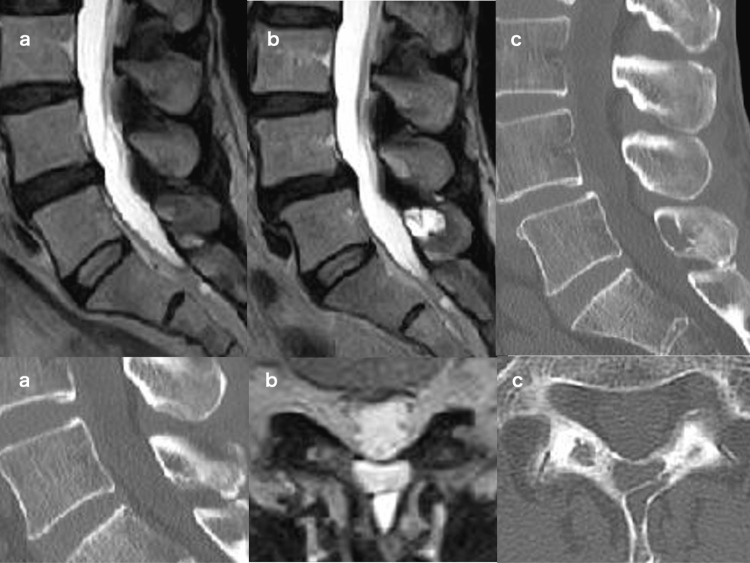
Changes in lumbar spinal images (a) Initial lumbar MRI (upper) and 3D-CT (lower) showed no more obvious findings. (b) MRI at symptom exacerbation showed L4-5 stenosis and spondylolisthesis with HLF and L5 laminar T2WI hyperintensity. Sagittal plane (upper), axial plane (lower). (c) 3D-CT at symptom exacerbation showed intramedullary low density and cavitation in the L5 laminar. Sagittal plane (upper), (lower) MRI: magnetic resonance imaging; CT: computed tomography; HLF: hypertrophied ligamentum flavum; 3D: three dimensional
